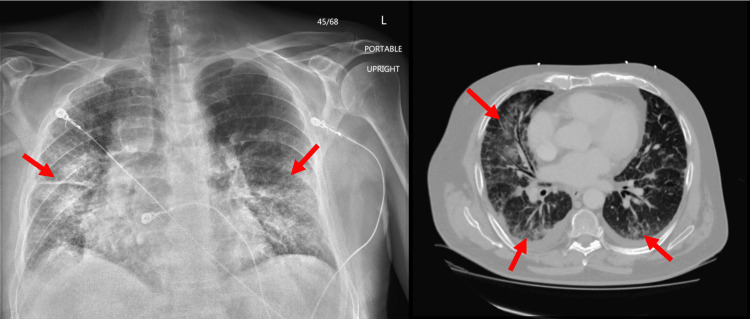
Left: Chest X-ray with nonspecific diffuse airspace opacity throughout the lungs bilaterally with sparing towards the left lung apex compared to chest X-ray 5 days prior. Right: CT chest with interval development of patchy ground-glass opacities bilaterally with baseline extensive fibrotic changes compared to CT chest 18 months prior.
